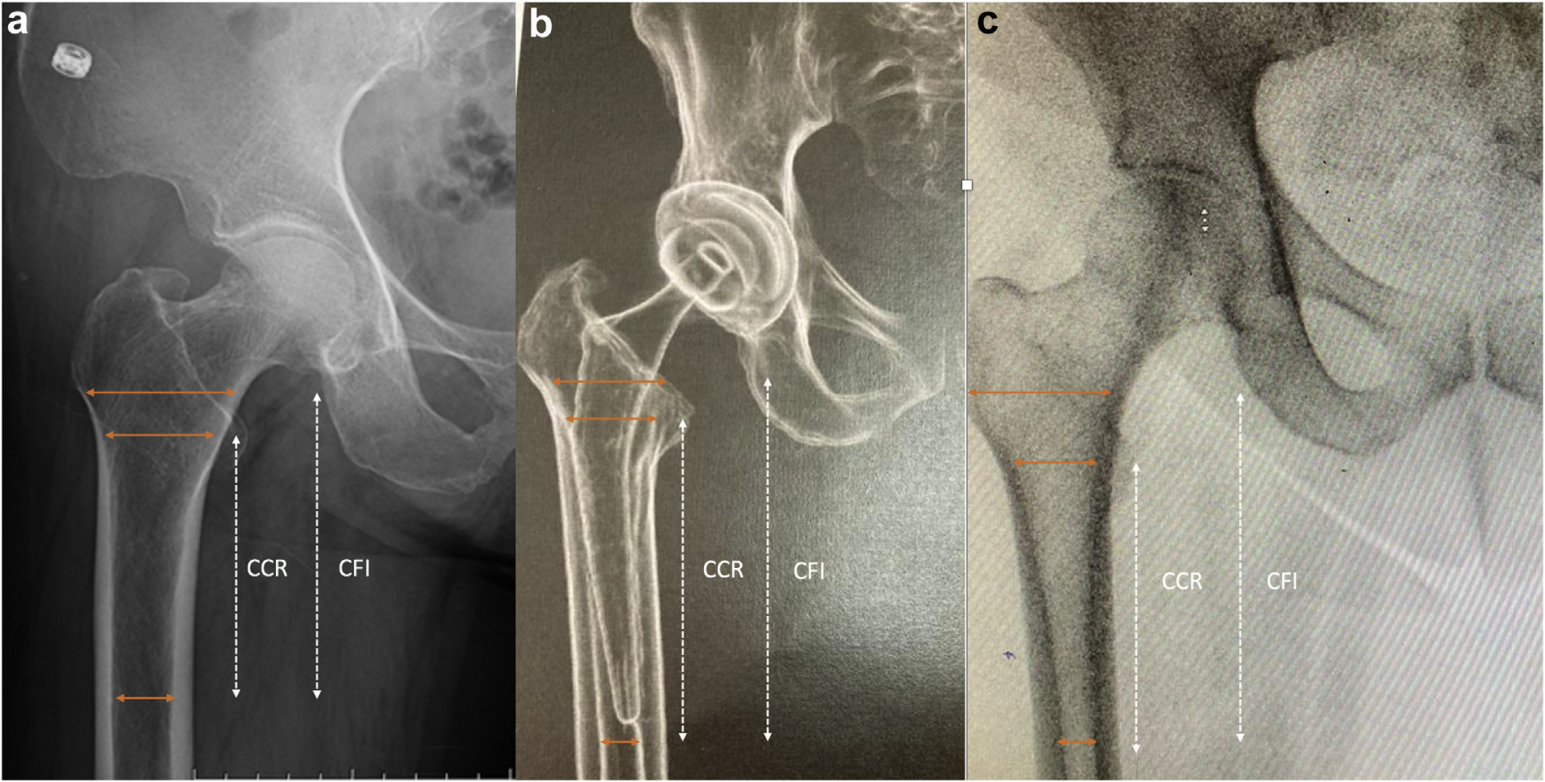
Radiograph control of a CFI < 3.0 (a, stovepipe-canal shape femur), (b) a CFI between 3.0 and 4.7 was normal canal shape, and (c) a CFI > 4.7 was considered a champagne-fluted canal shape.
